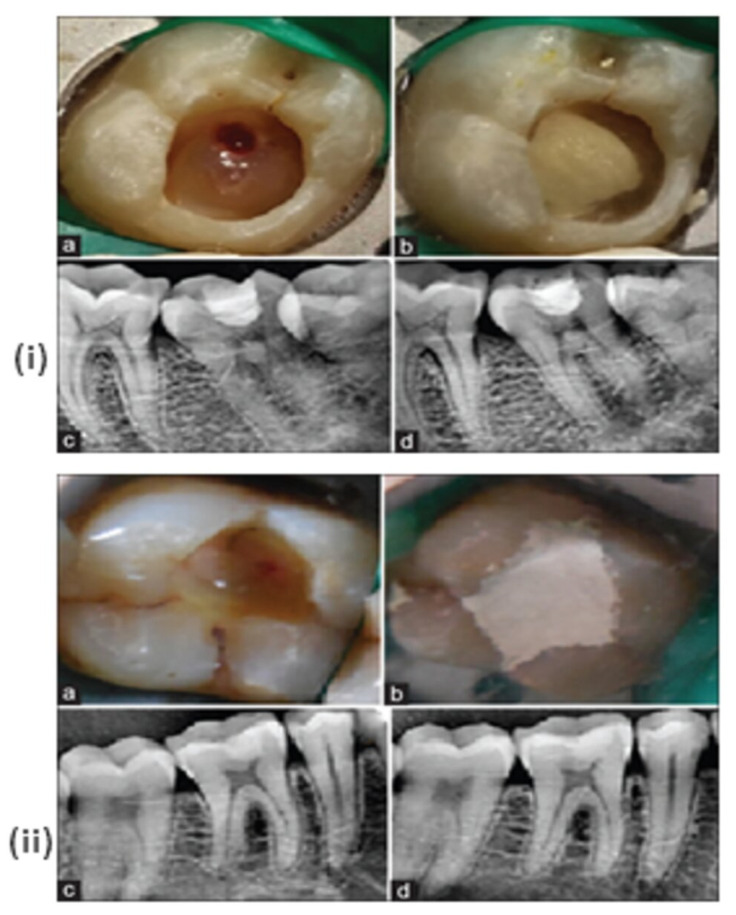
(i) (a) Exposure of pulp after caries excavation; (b) Placement of MTA over the exposed pulp; (c) Radiographic image showing placement of the MTA after pulp capping; (d) Follow-up radiograph after 6 months; (ii) (a) Exposure of pulp after caries excavation; (b) Placement of Biodentine over the exposed pulp; (c) Radiographic image showing placement of the Biodentine after pulp capping; (d) Follow-up radiograph after 6 months [215].
